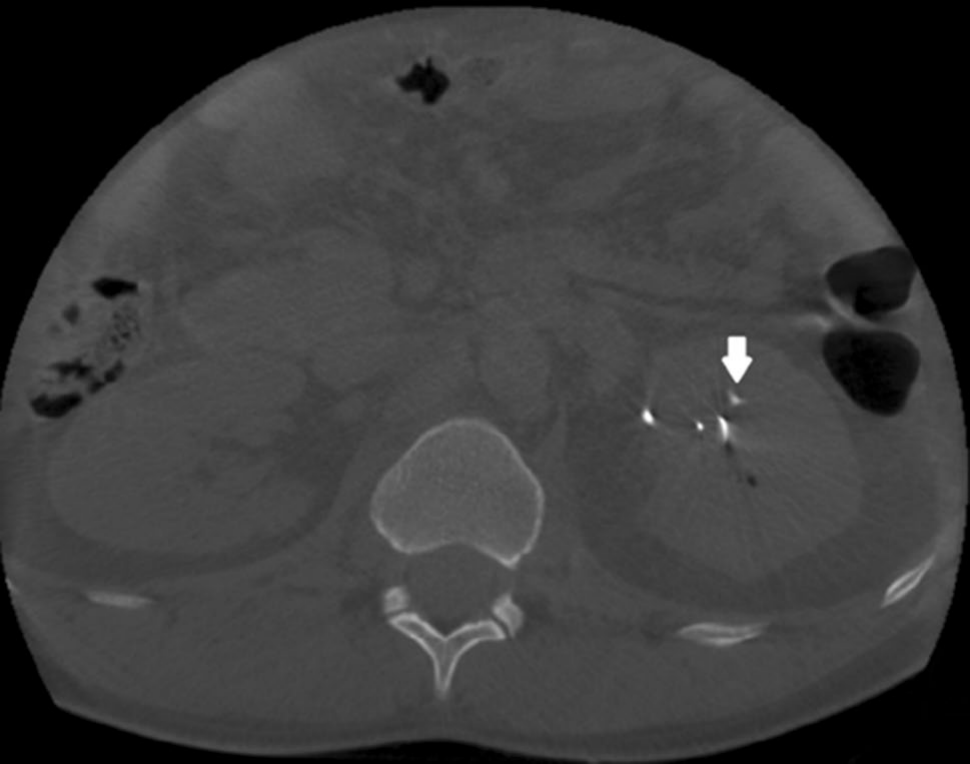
Example of an intraoperative CBCT-scan. The white arrow indicates a residual fragment that was extracted after acquiring the CBCT-images. The other white structures represent the occlusion catheter in the ureter and the safety wire
